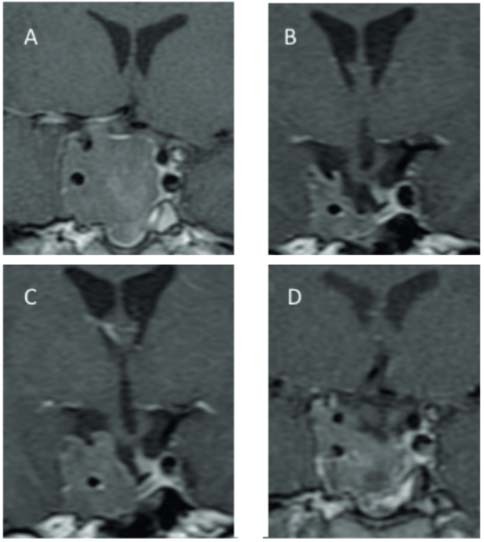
Sellar magnetic resonance imaging (T1 weighted after gadolinium enhancement) showing the growth of a residual tumor located mainly in the right cavernous sinus during pegvisomant treatment, that was sustained after drug withdrawal: (A) at diagnosis; (B) before pegvisomant treatment; (C) during pegvisomant treatment; (D) six months after pegvisomant withdrawal.
